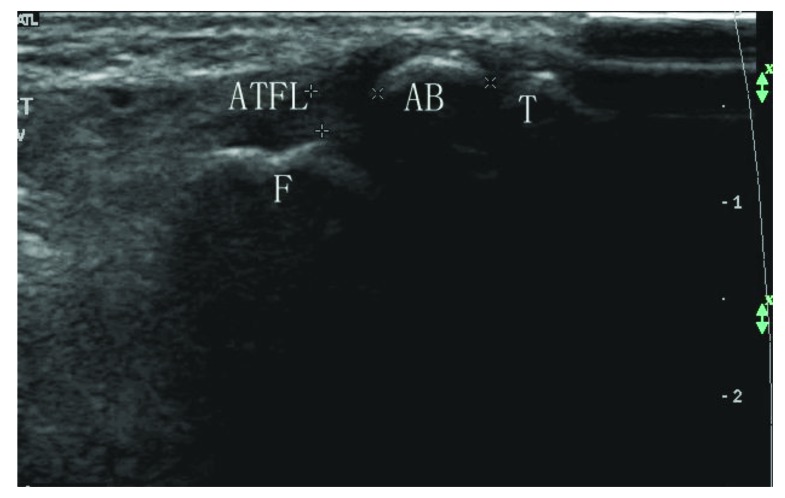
Sonogram shows a hyperechoic shadow (diameter > 0.5cm) in the remanent ligament at the fibular side (type IIC). ATFL: anterior talofibular ligament; F: fibula; T: talus; AB: avulsion bone
